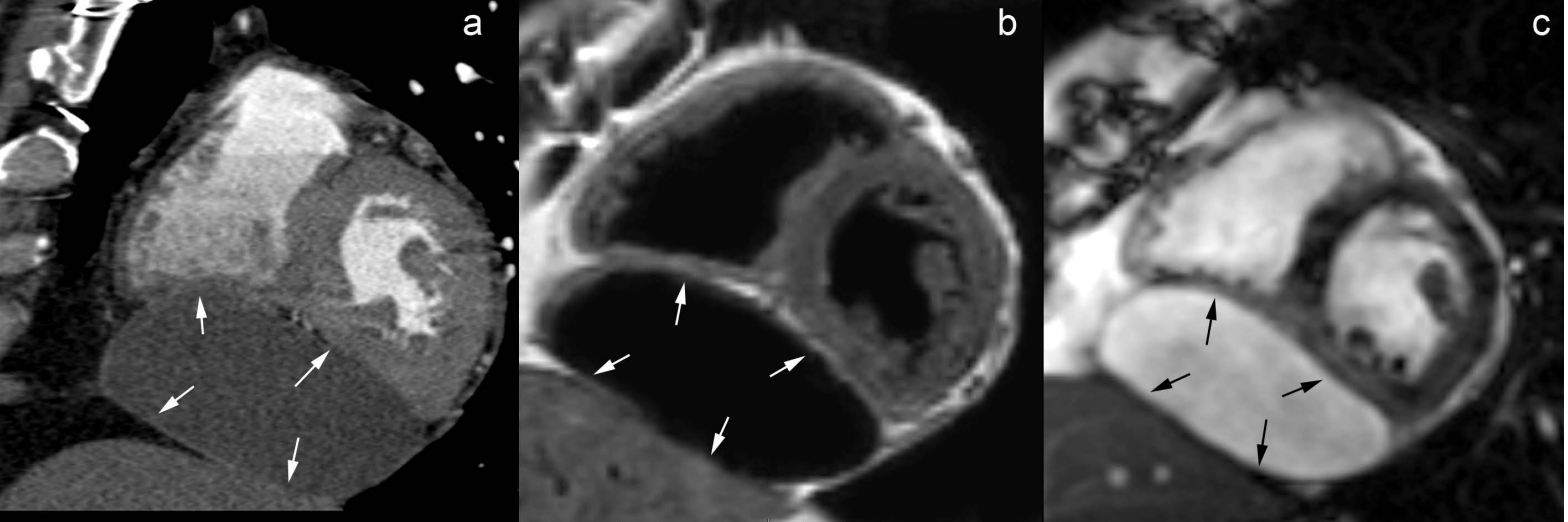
Loculated pericardial effusion with cardiac compression. Computed tomography (a), T1-weighted spin-echo CMR (b) and balanced SSFP CMR (c). All images shown in cardiac short-axis. Large oval-shaped pericardial effusion (arrows), inferiorly located to the heart. Note the compression on RV and LV.
